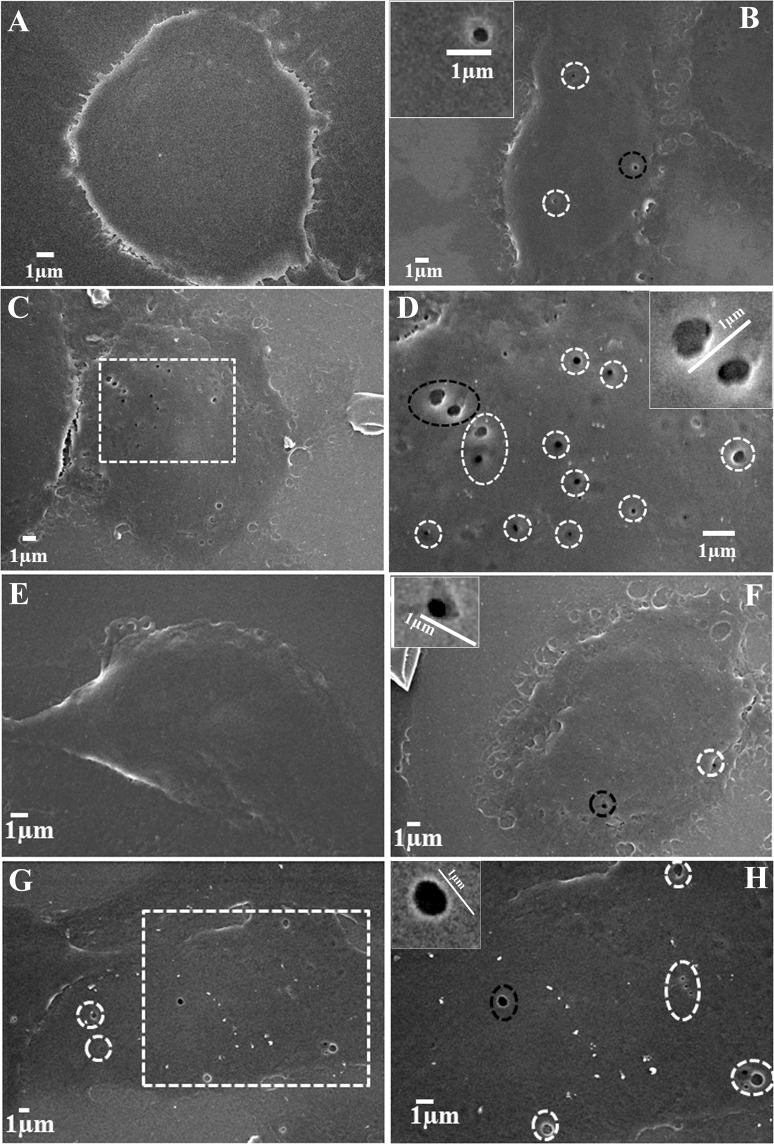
Sonopores developed in the plasma membrane of cells after treatment with ultrasound in the presence and absence of MB (A) untreated MDA MB 231 cells (B) ultrasound treatment in absence of MB, dotted circles show pores. The black circled pore is shown in the inset (C) ultrasound treatment in the presence of MB, a magnified view of the dotted area is shown in the image (D). (D) Dotted circles surround pores and black circled pore is shown in the inset. B16F10 melanoma cells (E) untreated (F) treated with only ultrasound, (G) treated with ultrasound in presence of MB; the dotted area is magnified in image H, (H) dotted circles surround pores and black dotted circle pore is shown in the inset.
